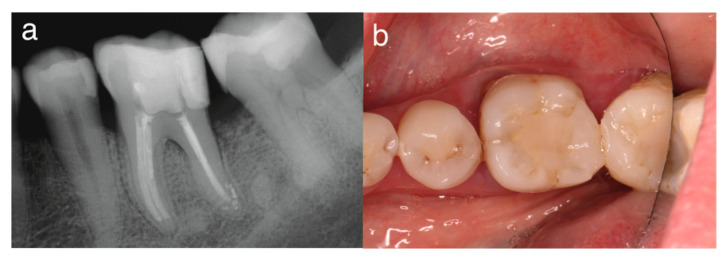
(a) Periapical radiograph and (b) occlusal view at 36-month follow-up.
